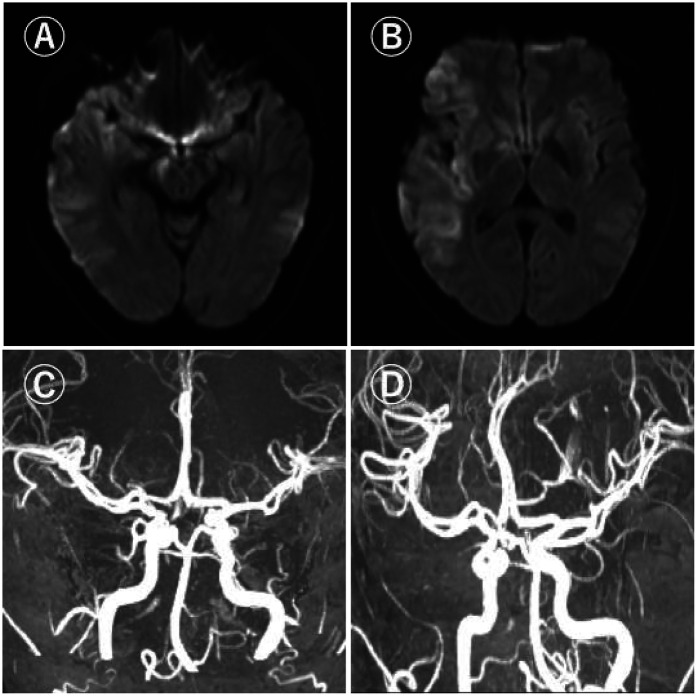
Postoperative magnetic resonance imaging.
